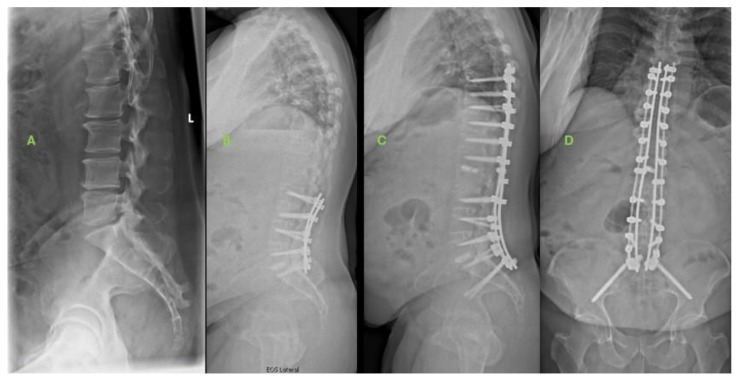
Example of SIJP+ patient. (A) Baseline radiographs of 59-year-old male with preoperative diagnoses of spinal stenosis, spondylosis, loss of lumbar lordosis, bilateral radiculopathy, and mild sacroiliac joint pain. (B) Six-week postoperative radiographs. The patient underwent L2-S1 anterior lumbar interbody fusion and L2-S1 posterior spinal fusion. Symptoms initially improved, but the patient developed progressive SIJ and back pain due to adjacent-segment pathology with proximal junctional kyphosis and thoracic stenosis and myelopathy. The patient subsequently underwent T10-L2 posterior spinal fusion with SIJ fusion 17 months after the index surgery. (C,D) Anteroposterior and lateral radiographs at 2 years post index surgery. SIJ symptoms resolved, with improvement in disability but still some residual activity-dependent back pain.
